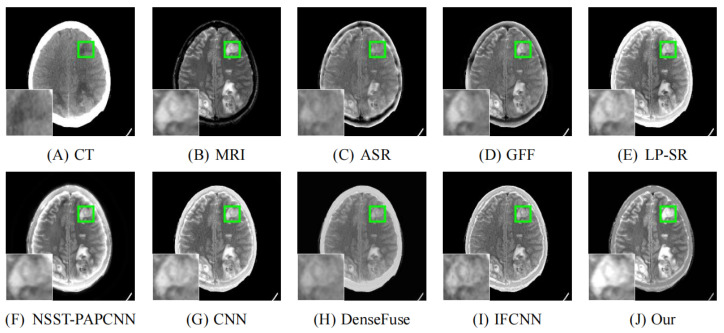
Fusion images of “Sarcoma-17” by various methods. From (A) to (J): CT, MRI, ASR, GFF, LP-SR, NSST-PAPCNN, CNN, DenseFuse, IFCNN, Our proposed method.
